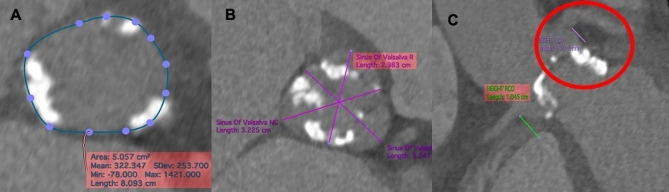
TAVR planning with Cardiac CT. (A) Aortic annulus with severe calcification, (B) Aortic valve with tricuspid morphology, (C) with severe calcification predominantly in the left sinus that extends to the LV outflow tract
